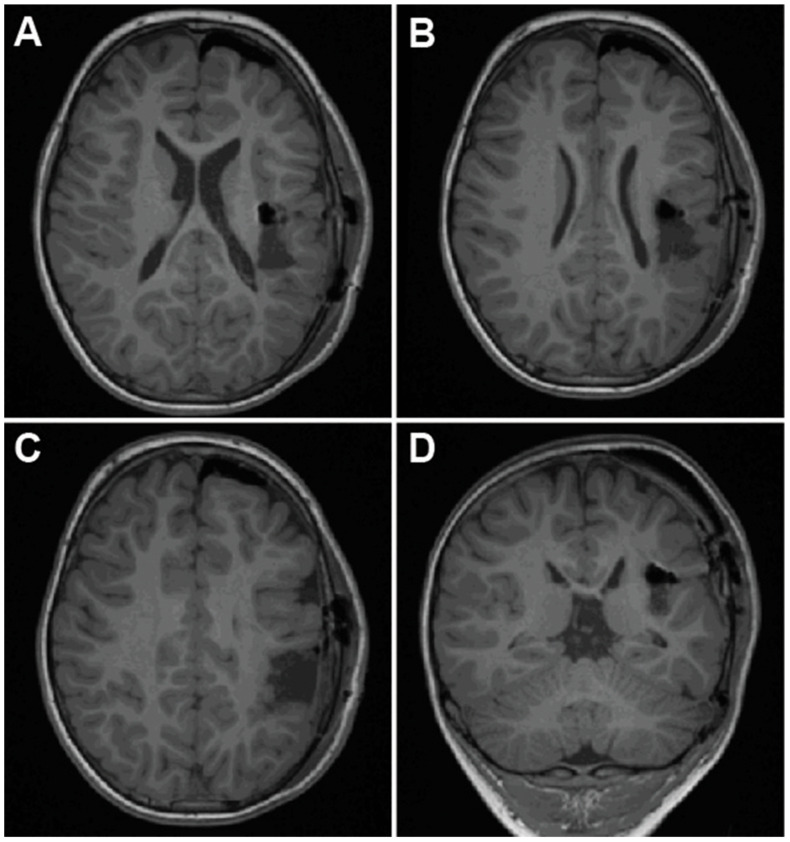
Axial (A–C) and coronal (D) postoperative T1-weighted MRI for Case 3 demonstrating resection cavity.
